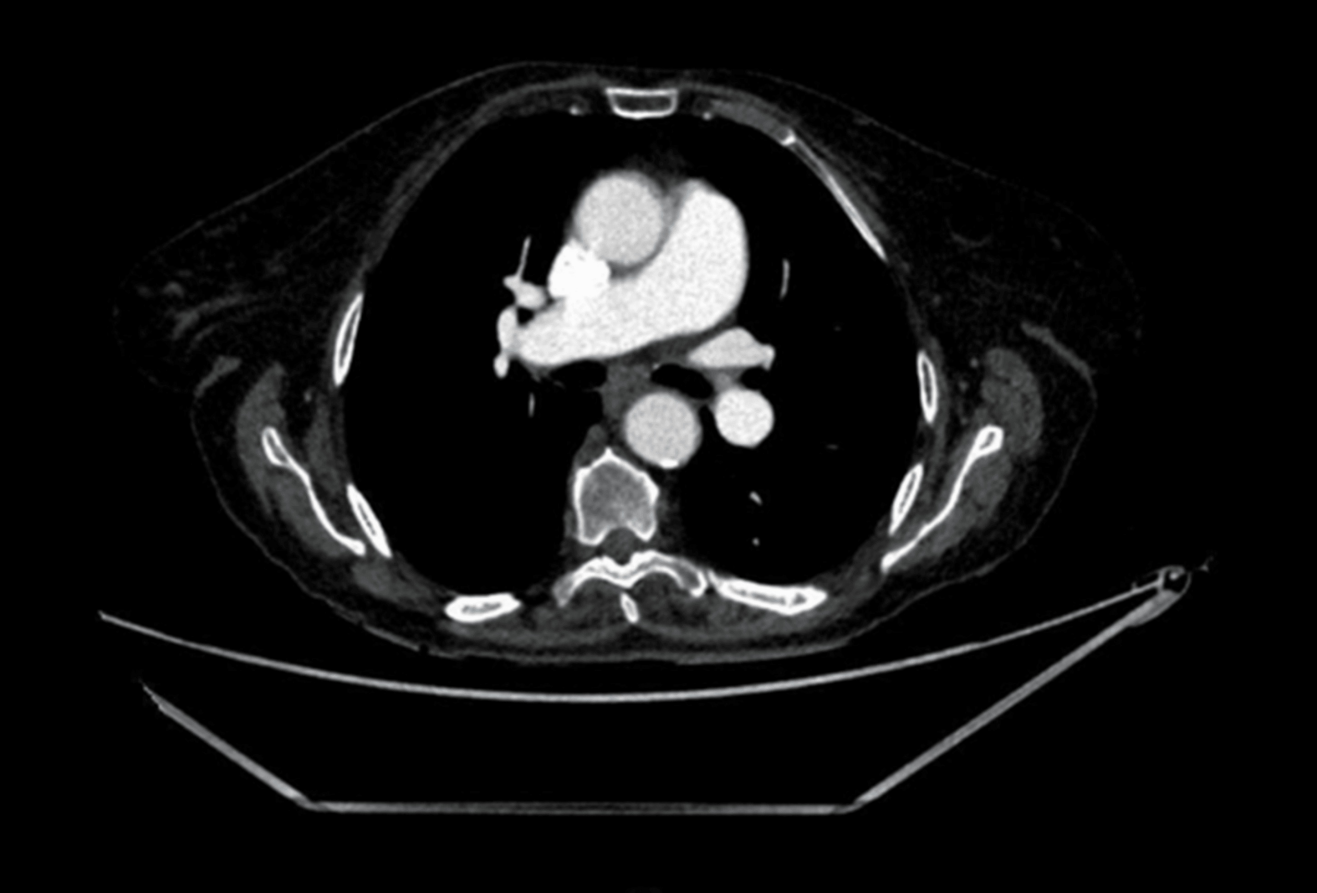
Contrast-enhanced CT angiogram demonstrating no evidence of large-vessel occlusion or alternative vascular cause of visual loss
